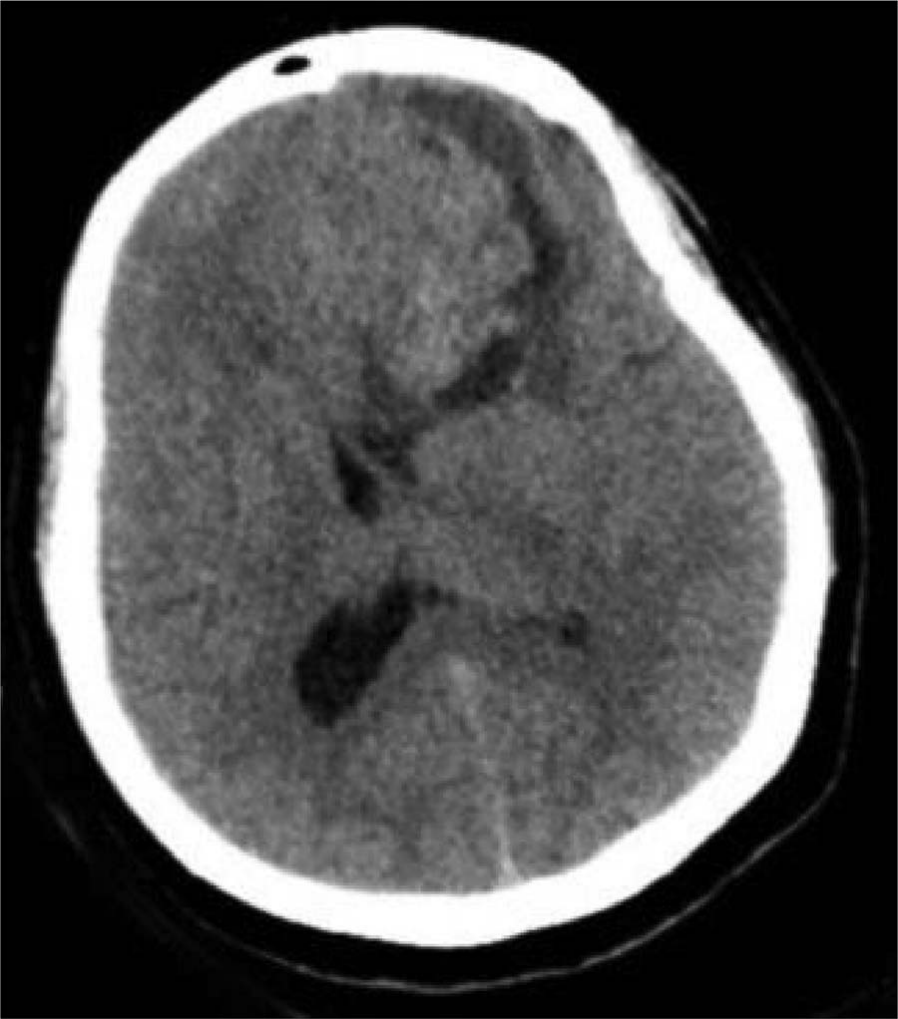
Preoperative CT brain without contrast (axial view) showing a well-circumscribed mass in the right frontal lobe resting on the falx cerebri, causing leftward midline shift with associated vasogenic edema and mass effect.
